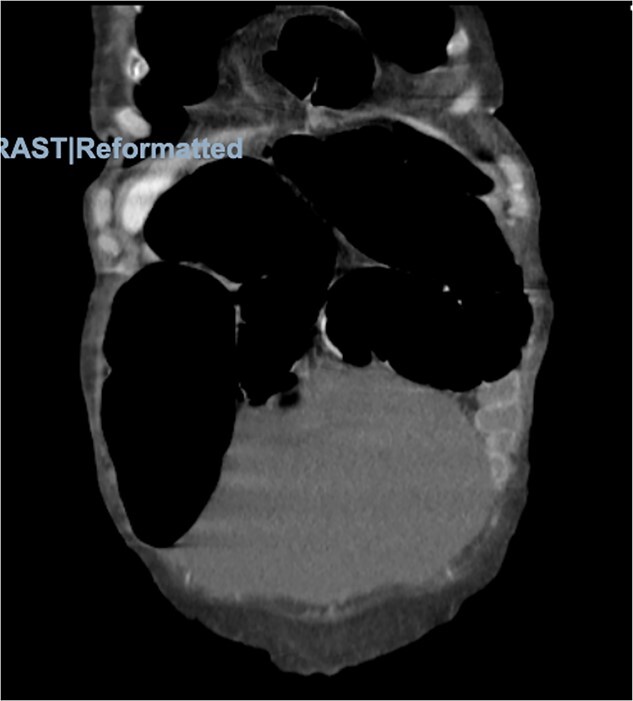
Morgagni hernia seen on CT abdomen and pelvis with contrast.
